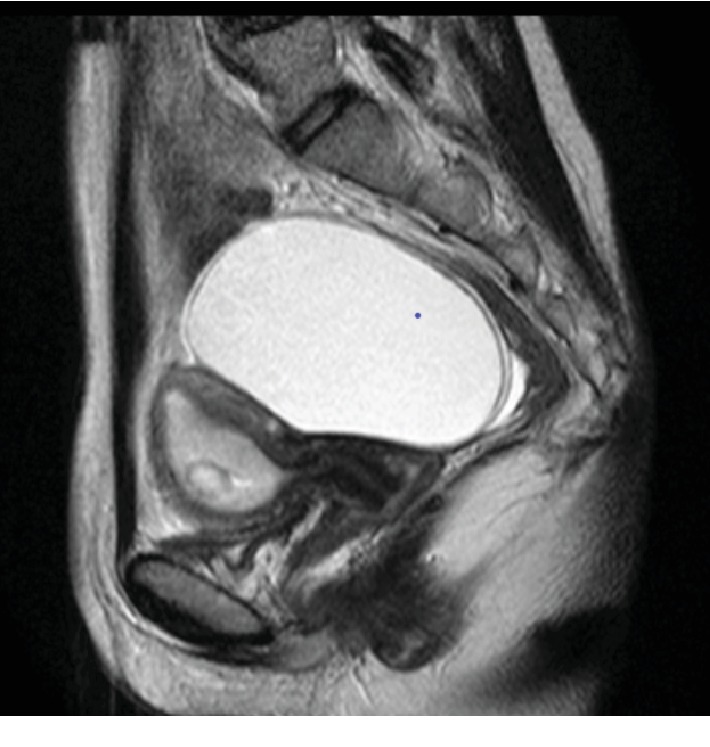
Sagittal T2-weighted MRI examination showed the possibility of benign ovarian cyst.
